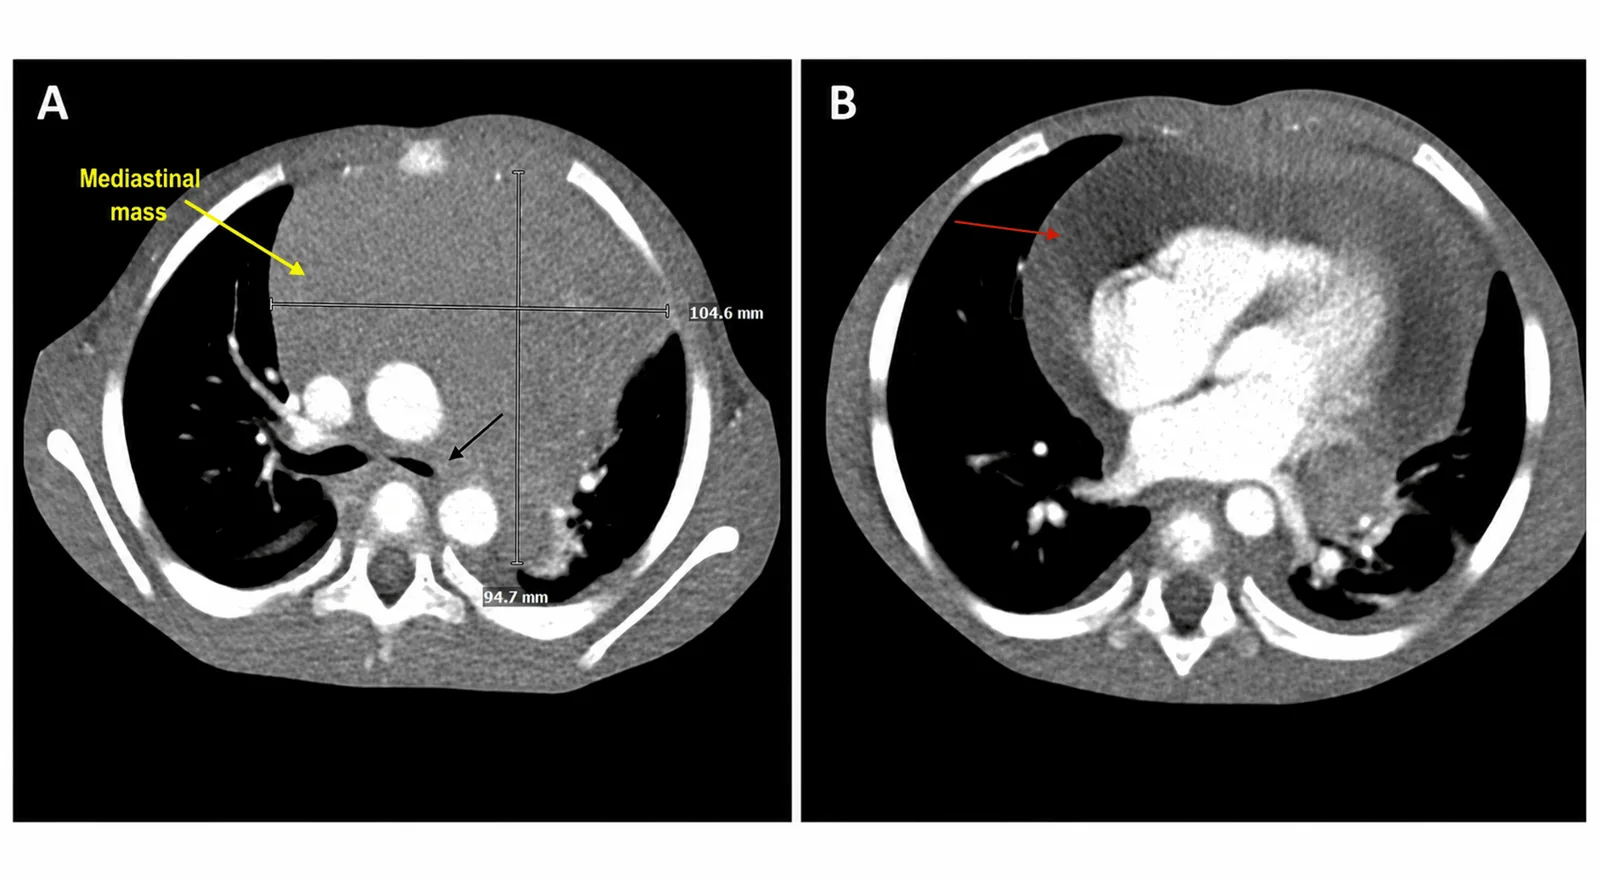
Chest CT-scan (A) Showing mediastinal mass (yellow arrow), mass causing compression of left lower bronchus (black arrow), and (B) showing pericardial effusion (red arrow).
